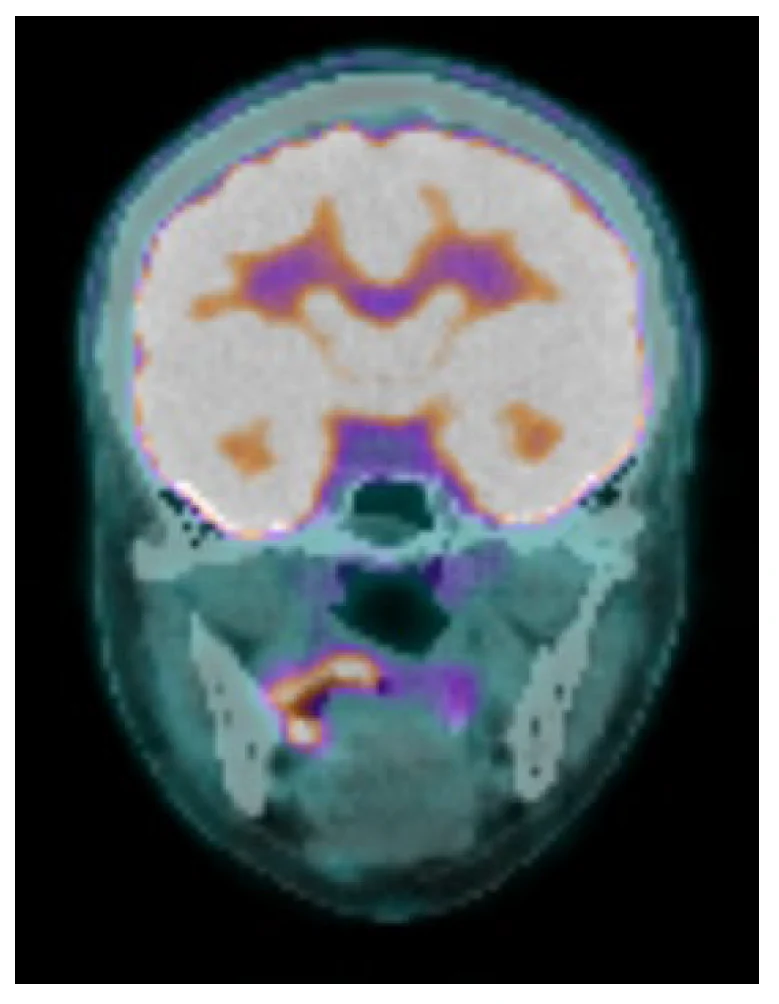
Coronal fluorodeoxyglucose (FDG) positron emission tomography–computed tomography (PET–CT) demonstrating focal increased metabolic activity in the right tonsillar region, corresponding to the suspected primary site, with associated metabolically active cervical lymphadenopathy.
